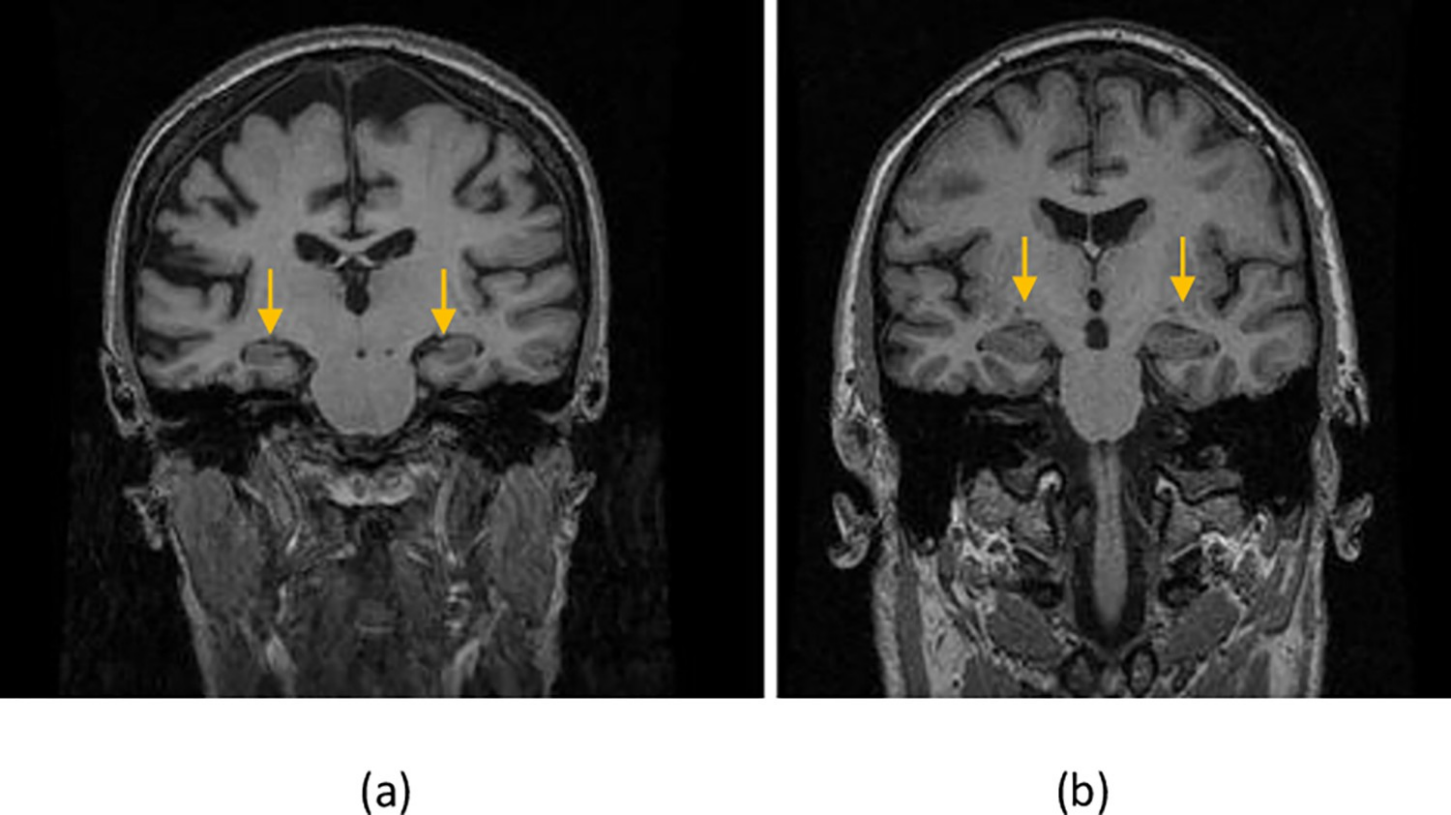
Coronal T1 weighted MRI images of (a) Alzheimer’s patient ID-201 and (b) normal patient ID-200 of the MIRIAD dataset. The FreeSurfer software divides the brain into left and right hemispheres and segments the medial temporal lobe into hippocampus, entorhinal, amygdala and para-hippocampal subregions.
